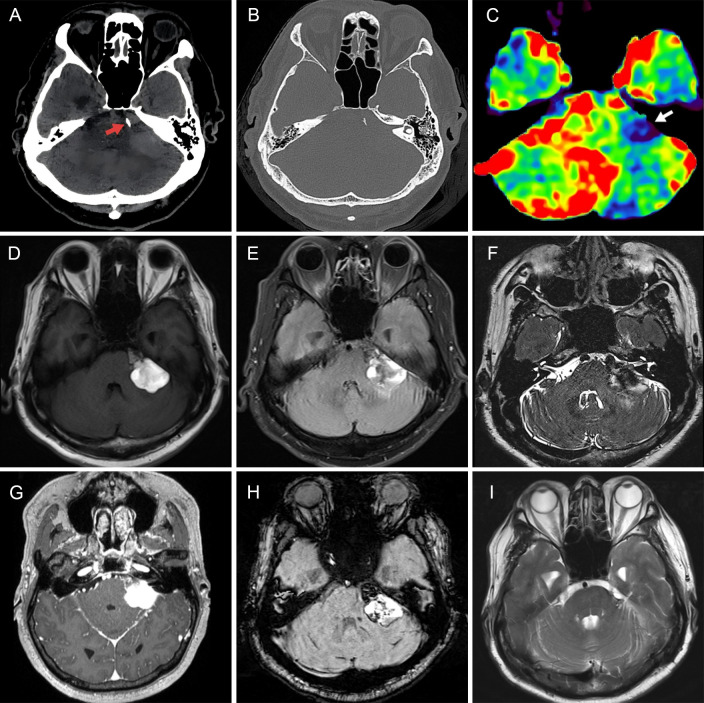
Preoperative and 8-month postoperative imaging. (A, B) Axial CT showing an irregular slightly hyperdense lesion with patchy calcifications in the left CPA (red arrows); no bony erosion. (C) CT perfusion indicating low perfusion in the lesion (white arrow). (D–F) MRI showing a 24 × 28 mm irregular CPA mass with hyperintensity on T1WI and mixed signals on T2-FLAIR and T2-SPACE. (G) Contrast-enhanced MRI demonstrating focal enhancement. (H) SWI revealing heterogeneous internal signals. (I) Follow-up MRI at 8 months confirming complete resection without recurrence.
